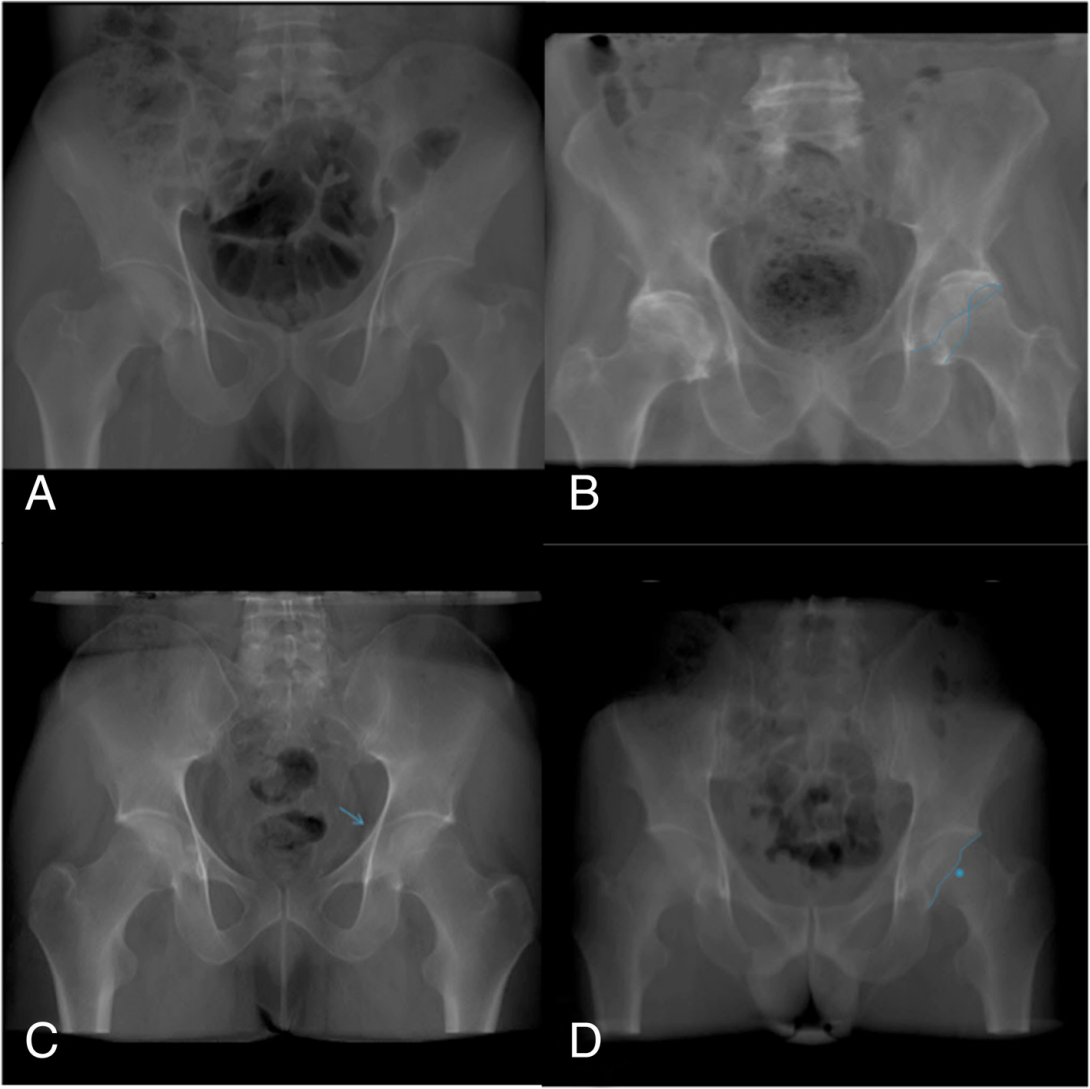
a Pelvis with no radiographical signs of acetabular retroversion. b Antero-posterior view, showing the cross-over sign on the left side. c Pelvis with the prominence of the ischial spine sign. The arrow indicates the ischial spine projecting into the lower pelvis on the left side. d Showing the posterior wall sign in the left hip. The posterior wall projects more medially then the center of the femoral head
